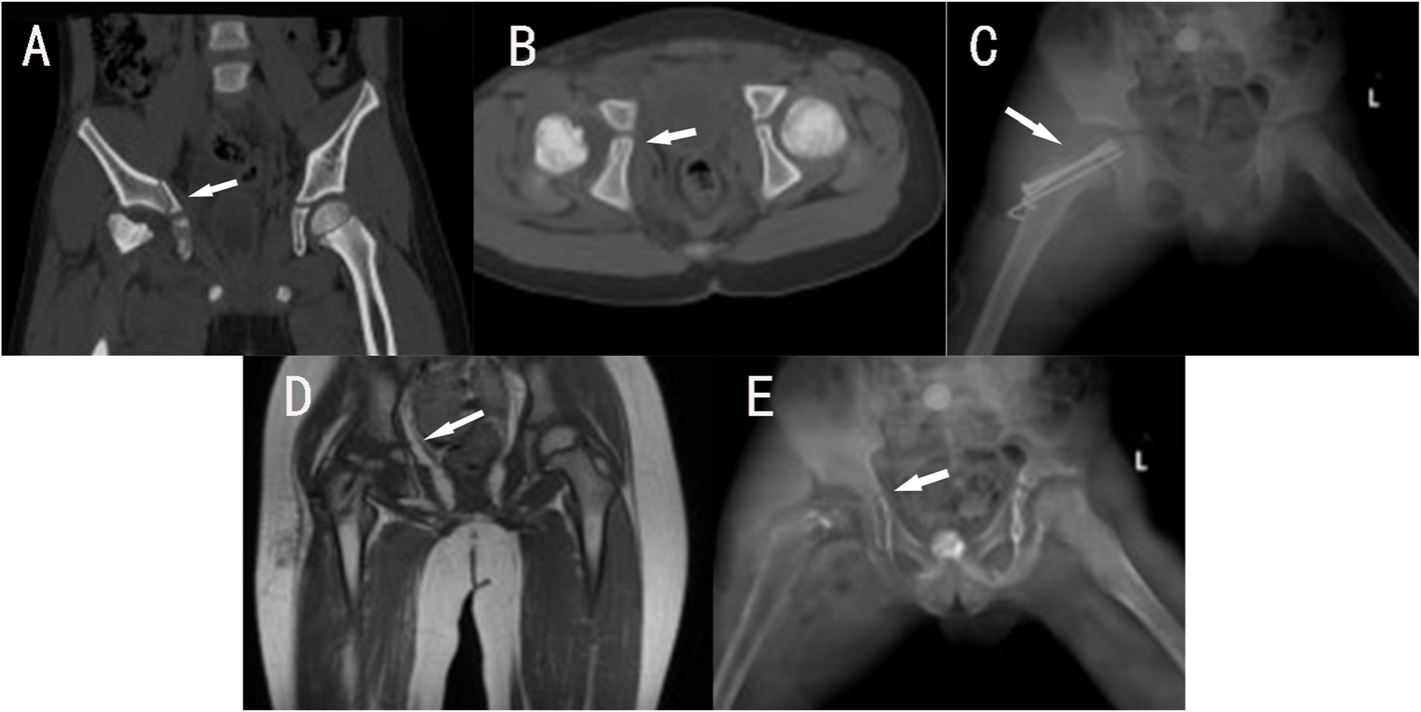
A 5.1-year-old boy(case E) was crushed by a car and caused a pelvic fracture involving right ilium and right acetabulum fractures, and a fracture of the left femur neck. CT showed a fracture line from the iliac metaphyseal through the triradiate cartilage to the ischiadic metaphyseal and was classified as type IV TCI (A, B) (white arrow). After the essential condition is stable, the right femur neck was performed ORIF (C). One year later, MRI showed partial necrosis and collapse of the femoral head, with the triradiate cartilage had no bone bridge formation (D). Four years after the operation, the patient had mild claudication with dysfunction such as squatting and stocking. X-ray showed slightly oblique acetabulum and necrosis of femoral head (E)
